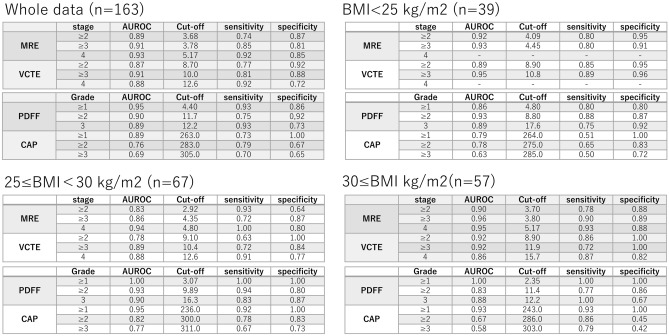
Comparison of the diagnostic accuracy of VCTE and MRI/MRE techniques to detect liver steatosis in patients with NAFLD presented by AUROCs. *MRE* magnetic resonance elastography, *MRI* magnetic resonance imaging, *VCTE* vibration-controlled transient elastography, *AUROC* area under the receiver operating curve.
